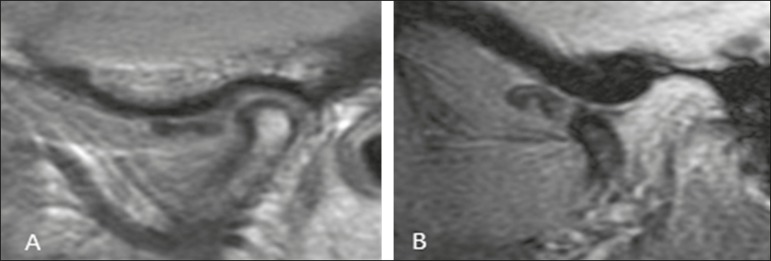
Sagittal MRI scans of the TMJ in a patient with DDWoR and disc deformity.
